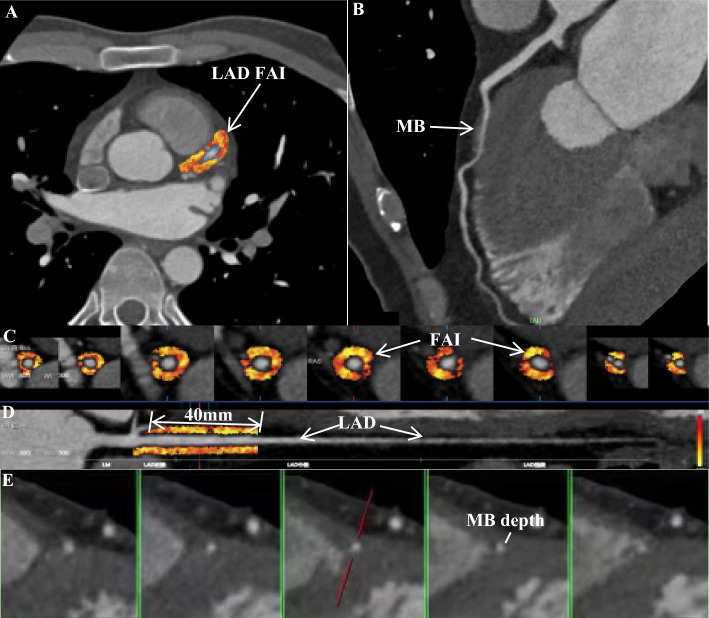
The method to quantify FAI and MB on cardiac computed tomography angiography images. (A) The axial plane image indicates the LAD and the FAI. (B) The axial plane image indicates the LAD and the MB. (C) Multiple axial plane images demonstrate the measurement of the FAI. (D) The FAI in the 40-mm proximal segment of the LAD is measured in a straightened view. (E) The axial plane image indicates the LAD and the MB depth. *FAI* fat attenuation index, *LAD* left anterior descending artery, *MB* myocardial bridging.
